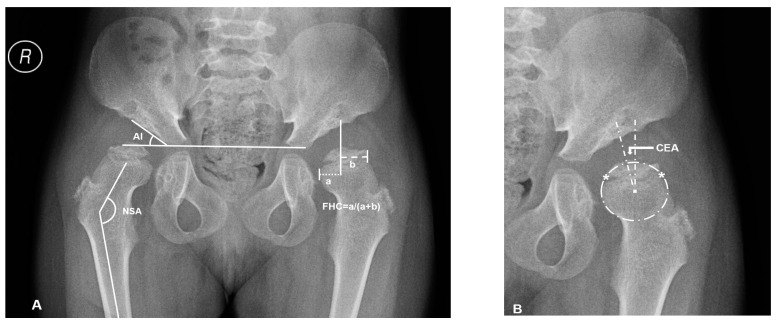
Overview of plain radiographic parameters in a patient with mucopolysaccharidosis (MPS) IVA (No.3: a 6.2-year-old female patient). (A) Right hip measurement of the acetabular index (AI) and neck shaft angle (NSA), left hip measurement of femoral head coverage (FHC): ‘a’ is the distance between osseous lateral margin of acetabulum and medial margin of femoral head; ‘b’ is the distance between osseous lateral margin of acetabulum and lateral margin of femoral head. (B) The method of Siddesh to define the center of incongruity head. Reference points ‘*’ are marked on the medial and lateral limits of the growth plate. A circle is marked connecting both the reference points, ensuring that the circle just touches the inner margin of the epiphysis but does not extend outside the femoral head. The center of this circle is marked, and then center-edge angle (CEA) is measured.
